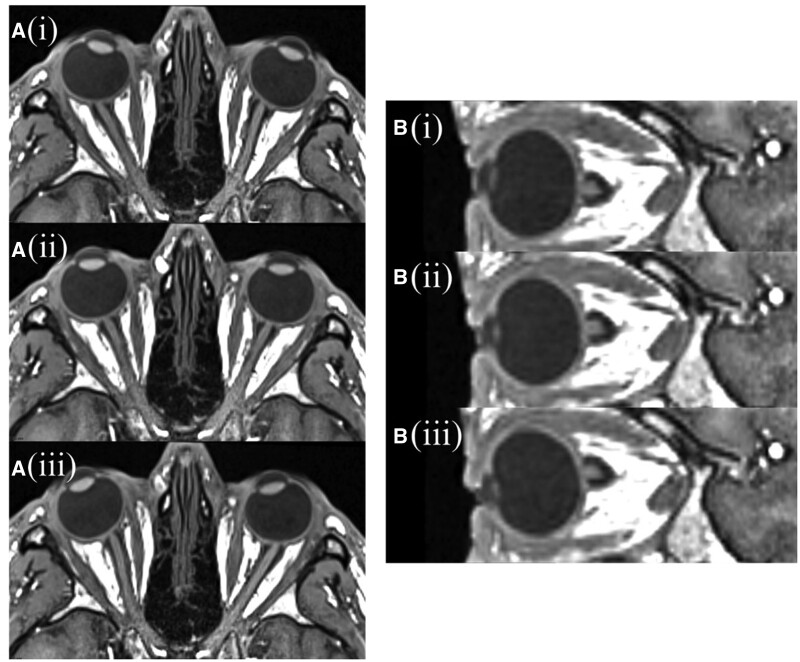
MRI images showing optic nerve motion: (A) axial scans with the volunteer looking (i) left, (ii) neutral, and (iii) right; and (B) coronal scans with the volunteer looking (i) up, (ii) neutral, (iii) down.
